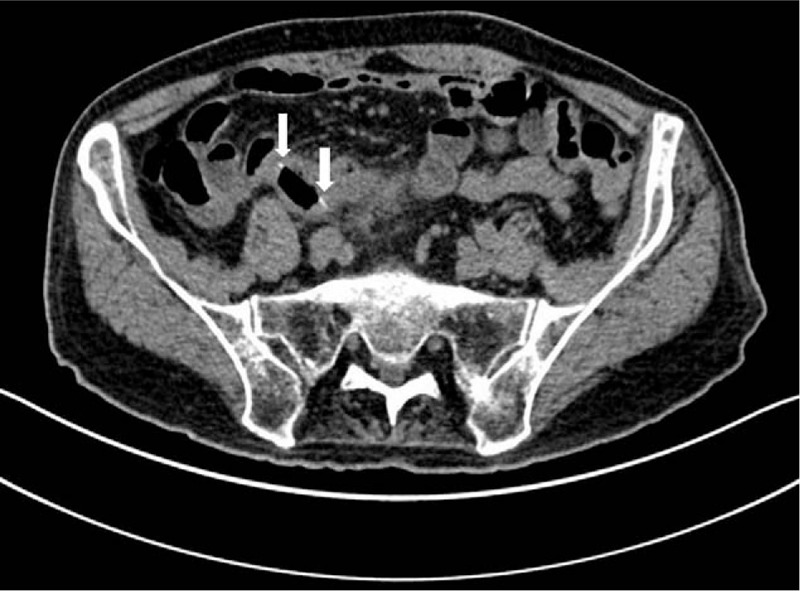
An additional axial CT image. An additional axial CT slice through the foreign body across the center of the pill packaging.
